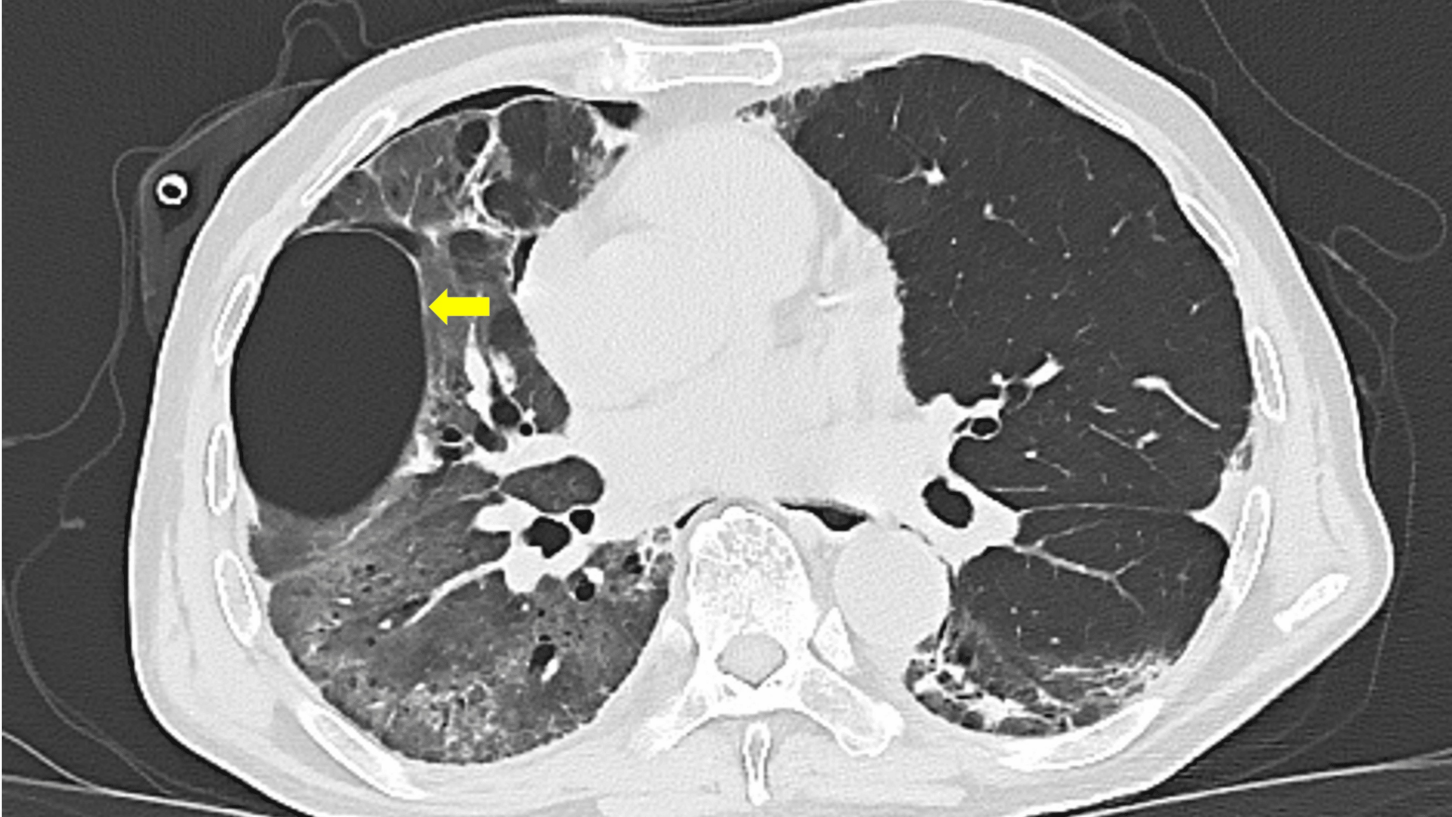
Follow-up chest CT after chest drainage showing a pneumatocele (arrow).
